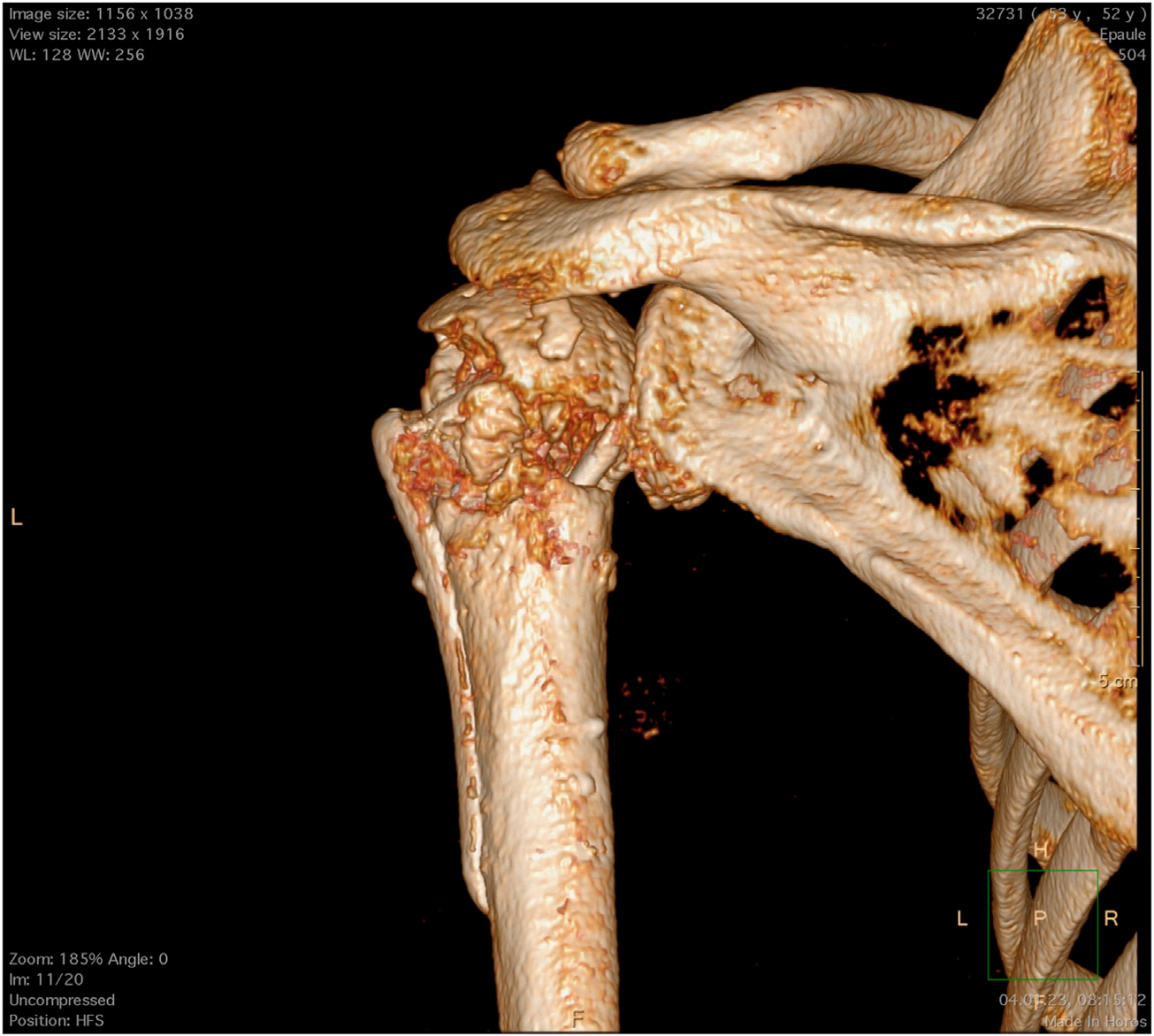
Three-dimensional computed tomography scan reconstruction of a left shoulder from a posterior point of view. A greater tuberosity deficiency can be noted.
